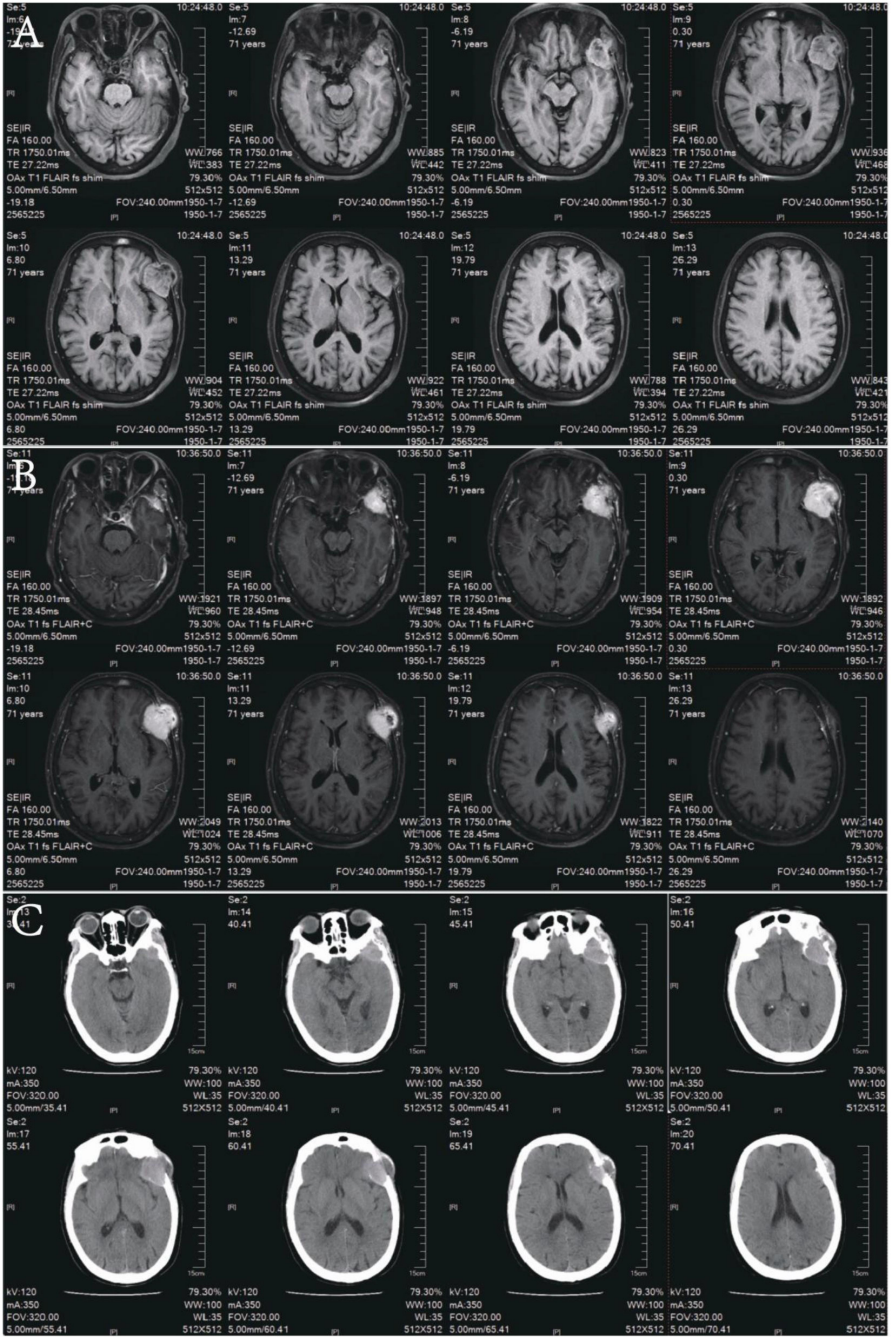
Pre-operative imaging of the patient. (A) T1-weighted MRI. (B) Contrast-enhanced MRI. (C) CT scan.
